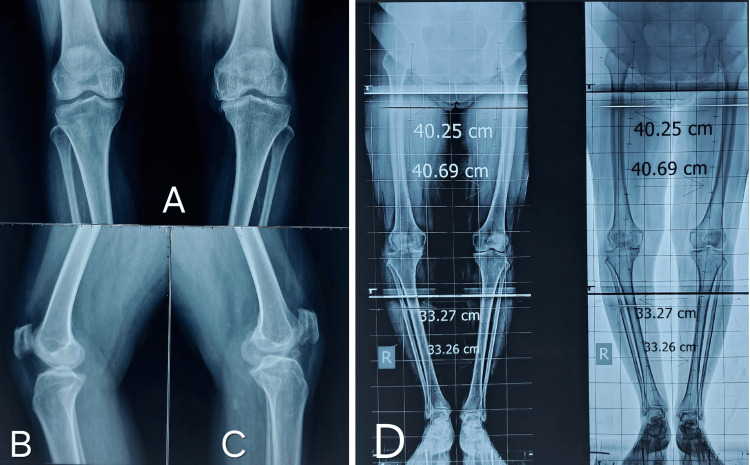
A) Radiograph showing anteroposterior view of the knee joint in standing weight bearing position. B, C) Radiograph showing lateral view of both knees. D) Standing orthoscannogram of both lower limb in weight bearing posture.
